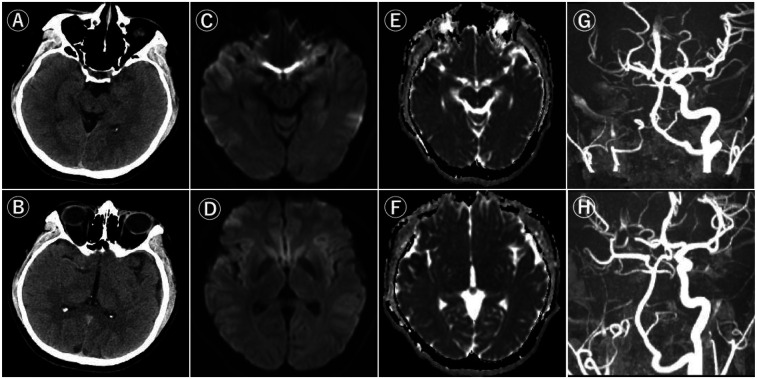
Computed tomography and magnetic resonance imaging.
